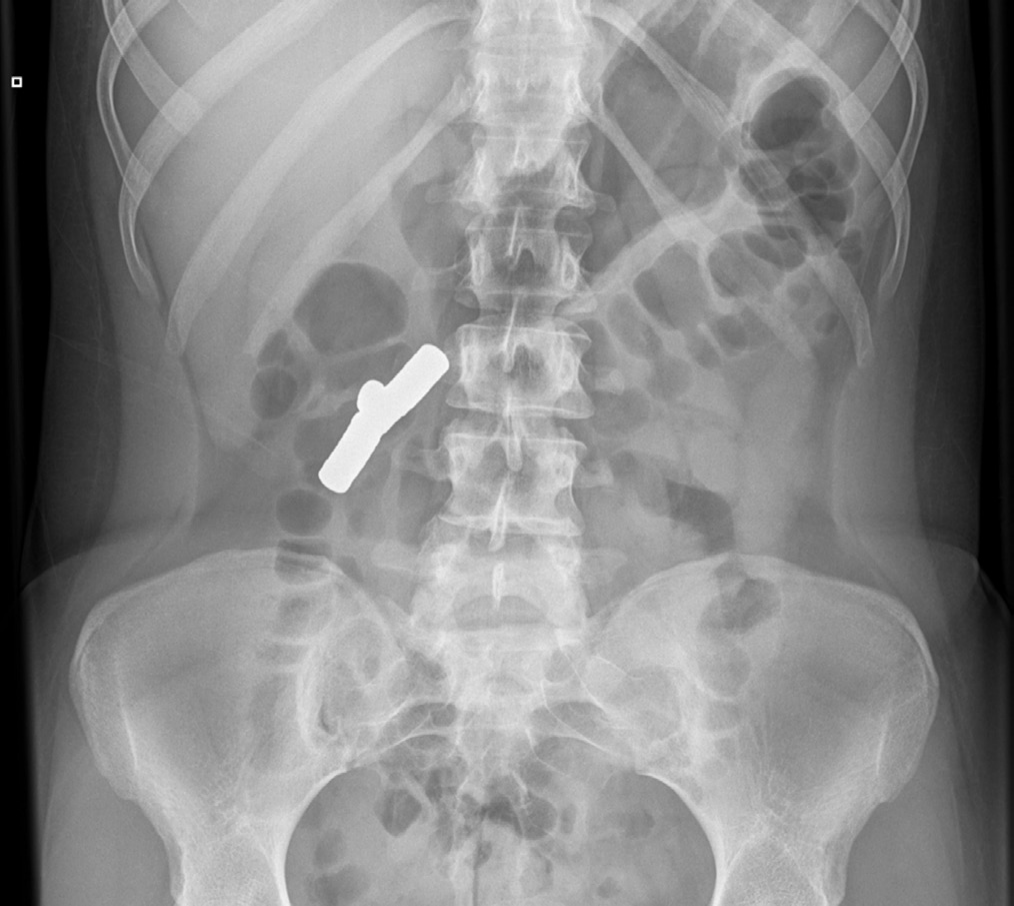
Abdominal radiograph showing the aggregation of button magnets in the right upper quadrant.
